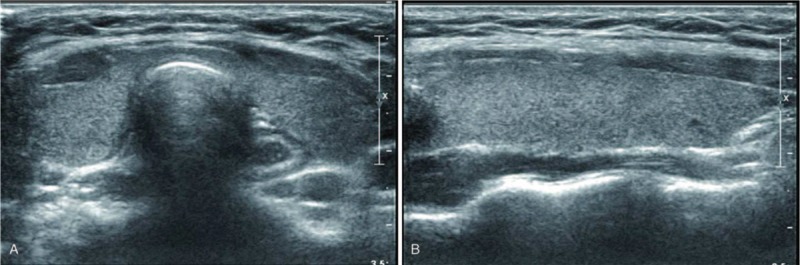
(A) Transverse and (B) longitudinal ultrasonographic images of Grade 1.
